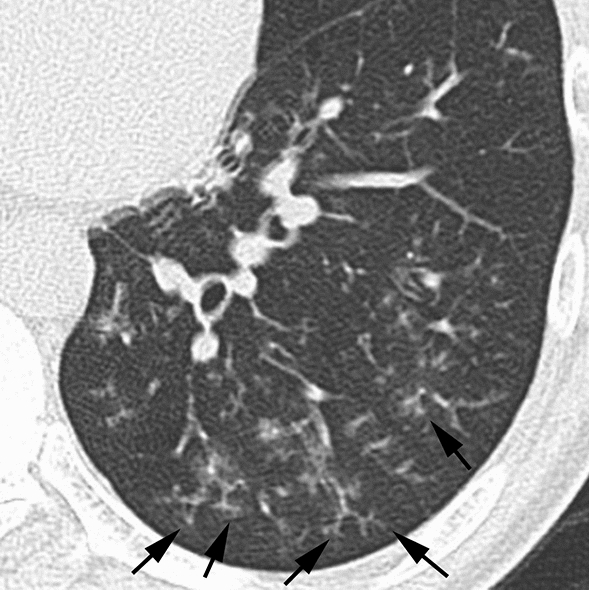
Infectious disease (bacterial pneumonia) in a 56-year-old male patient with acute myelogenous leukemia. HRCT image of the left lower lung showing nodules with a centrilobular distribution and TIB pattern (arrows). Note that ILS thickening is not evident
